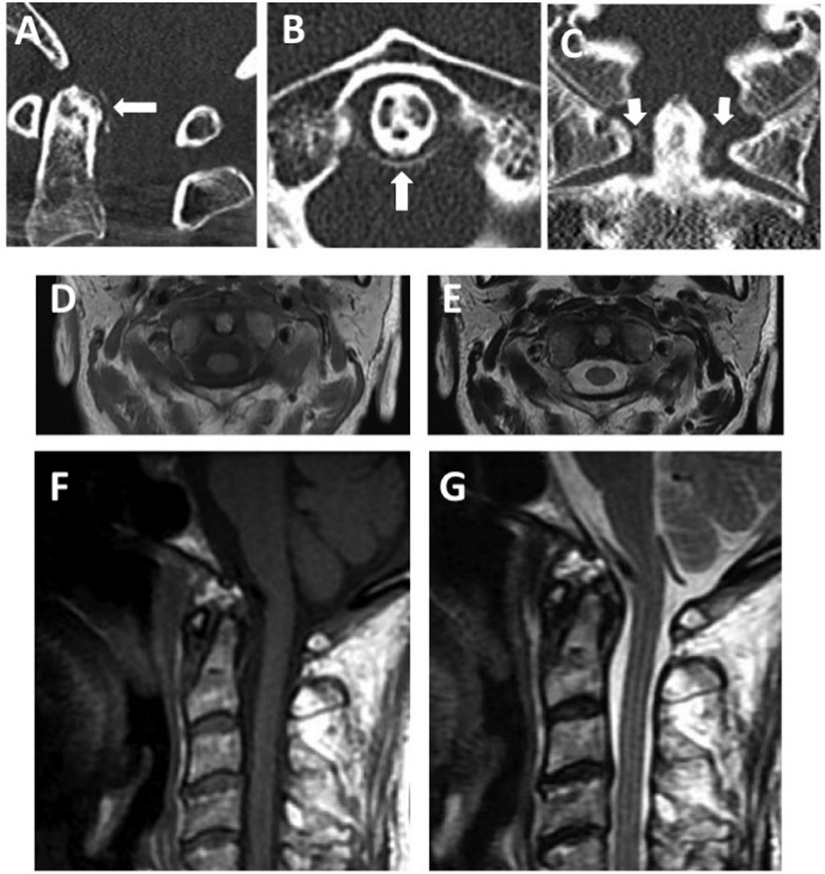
Images of crowned dens syndrome. (A) Cervical sagittal CT. (B) Cervical axial CT. (C) Cervical coronal CT. All cervical CT scans reveal calcified lesions around the dens. (D–G) Cervical T1-weighted imaging [(D) axial; (F) sagittal] and T2-weighted imaging [(E) axial; (G) sagittal] before treatment. A cervical MRI reveals no swelling or prevertebral edema.
